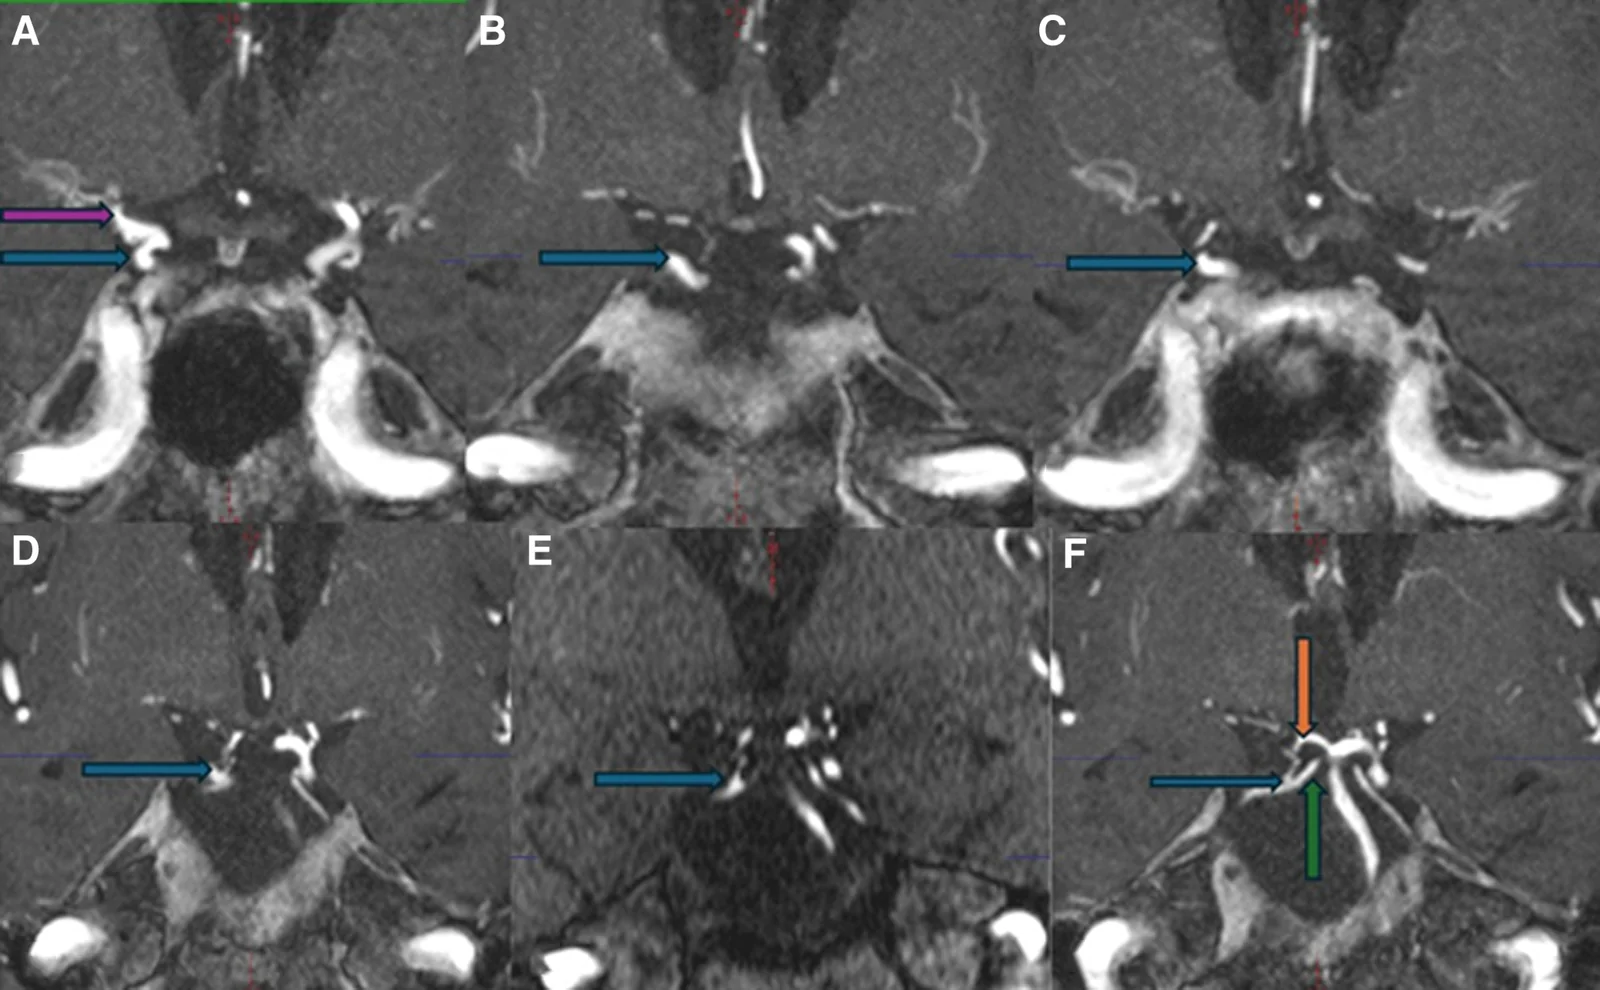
CTA coronal view slides reveal the course of the right posterior communicating artery (blue arrow) from the right internal carotid artery to its combination with the right superior cerebellar artery (purple arrow). Right posterior cerebral artery P1 (orange arrow). The right posterior communicating artery combines with the right superior cerebellar artery (green arrow).
